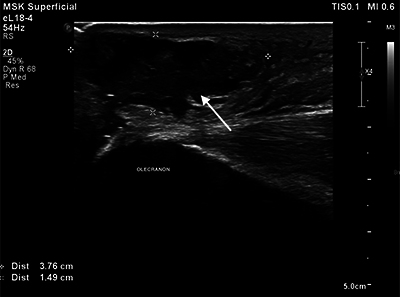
Abscess (arrow) situated adjacent to the olecranon on the ultrasound study.
